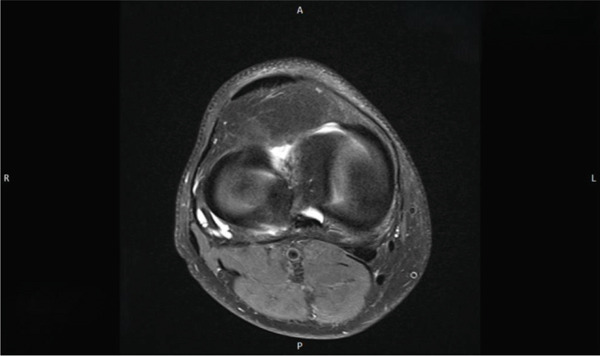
MRI of the right knee joint (axial view). In this imaging the "R" stands for "right side" and the "L" stands for "left side." Accordingly the letter "A" stands for "anterior" and the letter "P" stands for "posterior."
